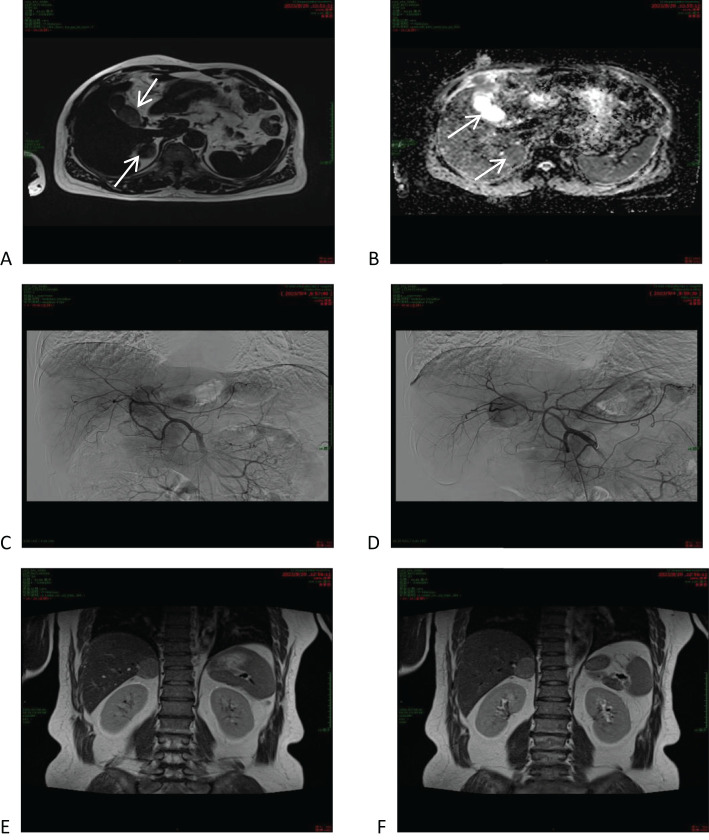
Magnetic resonance imaging (MRI) of the upper abdomen and hepatic arteriography. (A, B) MRI of the upper abdomen and hepatic arteriography showed that the right posterior lobe of the liver was slightly low-signal in T1WI and relatively high-signal in T2WI, and the lumen of the gallbladder was isosignal in T1WI and slightly high-signal in T2WI. (C, D) Hepatic arteriography shows irregularly disorganized tumor vessels proximal to the right hepatic artery. (E, F) CT showed that the mass was located in the lower part of the right posterior lobe of the liver, adjacent to the right adrenal gland.
